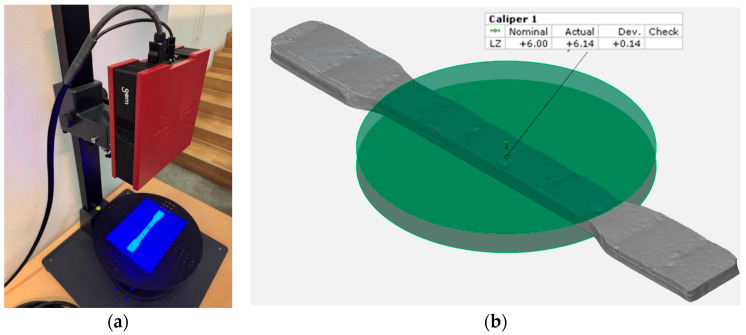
(a) 3D Scanning GOM; (b) ZEISS INSPECT Optical 3D.
